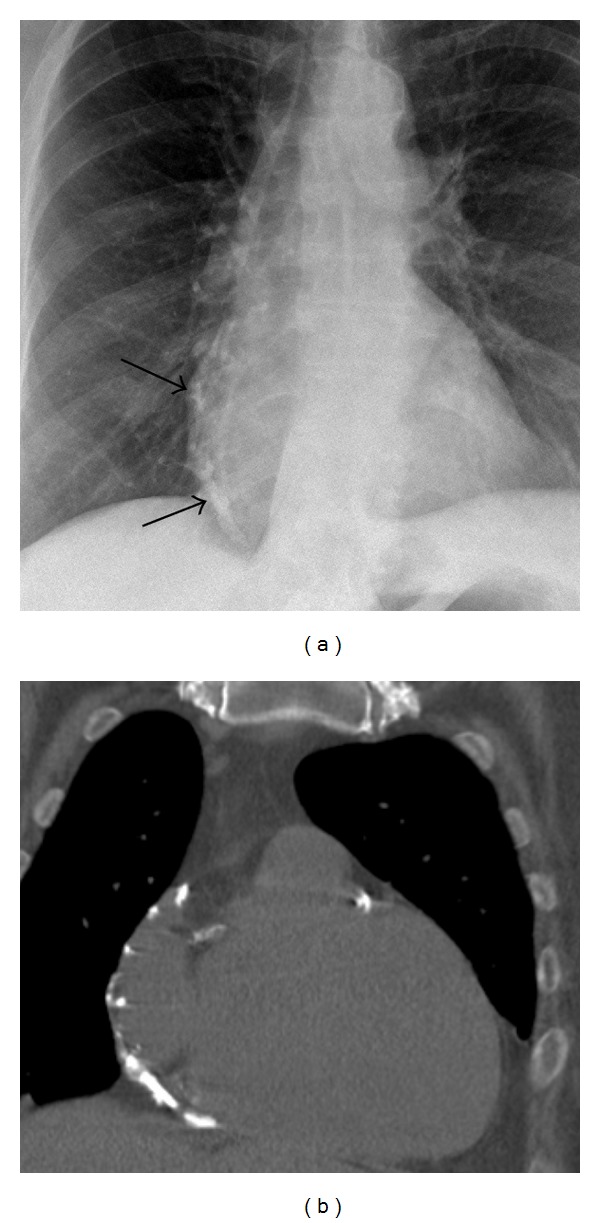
A 53-year-old woman with extensive cardiac history. (a) Chest radiograph and (b) coronal reconstructed CT image demonstrate dense calcification of the pericardium, predominantly on the right. With history of myocardial infarction, pericarditis, systemic lupus erythematosus, and end-stage renal disease, she has several independent possible explanations for her pericardial calcifications.
